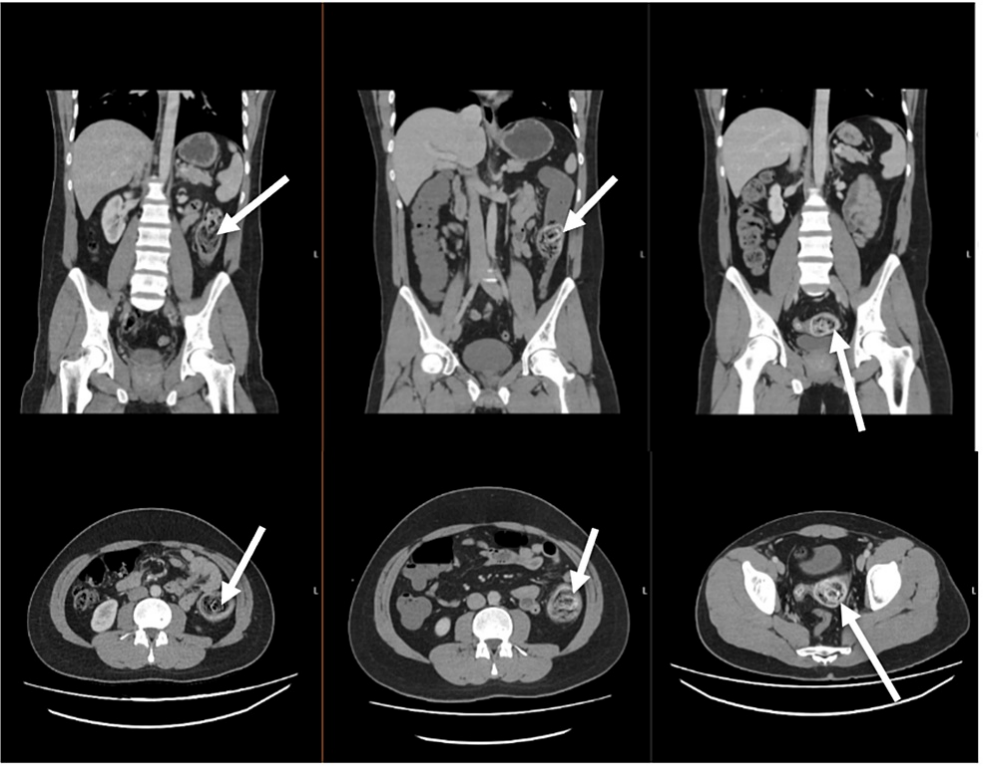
The patient's CT scans (case two) A set of coronal (top row) and transverse (bottom row) CT images of the abdomen and pelvis Images are shown in the order of the patient's presentation: April 2021 (left column), admission June 2023 (middle column), and after flexible sigmoidoscopy June 2023 (right column). The calcified faecolith can be seen migrating from the descending colon (left column) to the proximal sigmoid colon (middle column) and to the distal sigmoid colon (right column).
